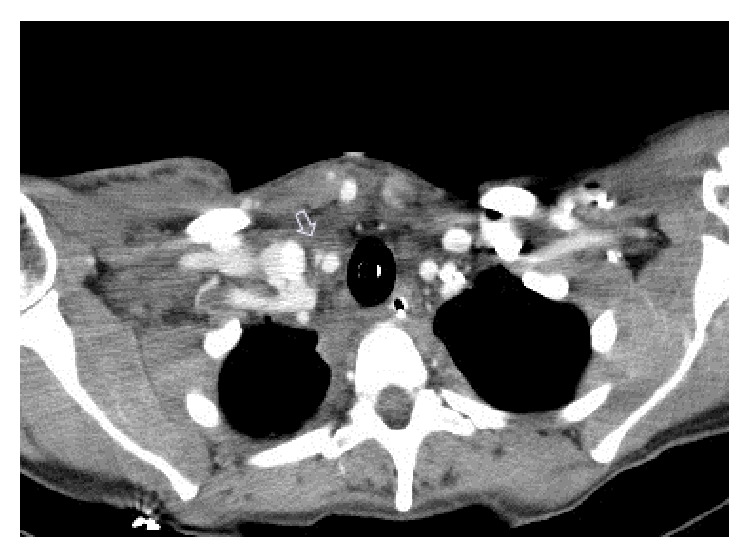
The initial computed tomography (CT) scan revealed a small dissection in the right common carotid artery (white arrow).
